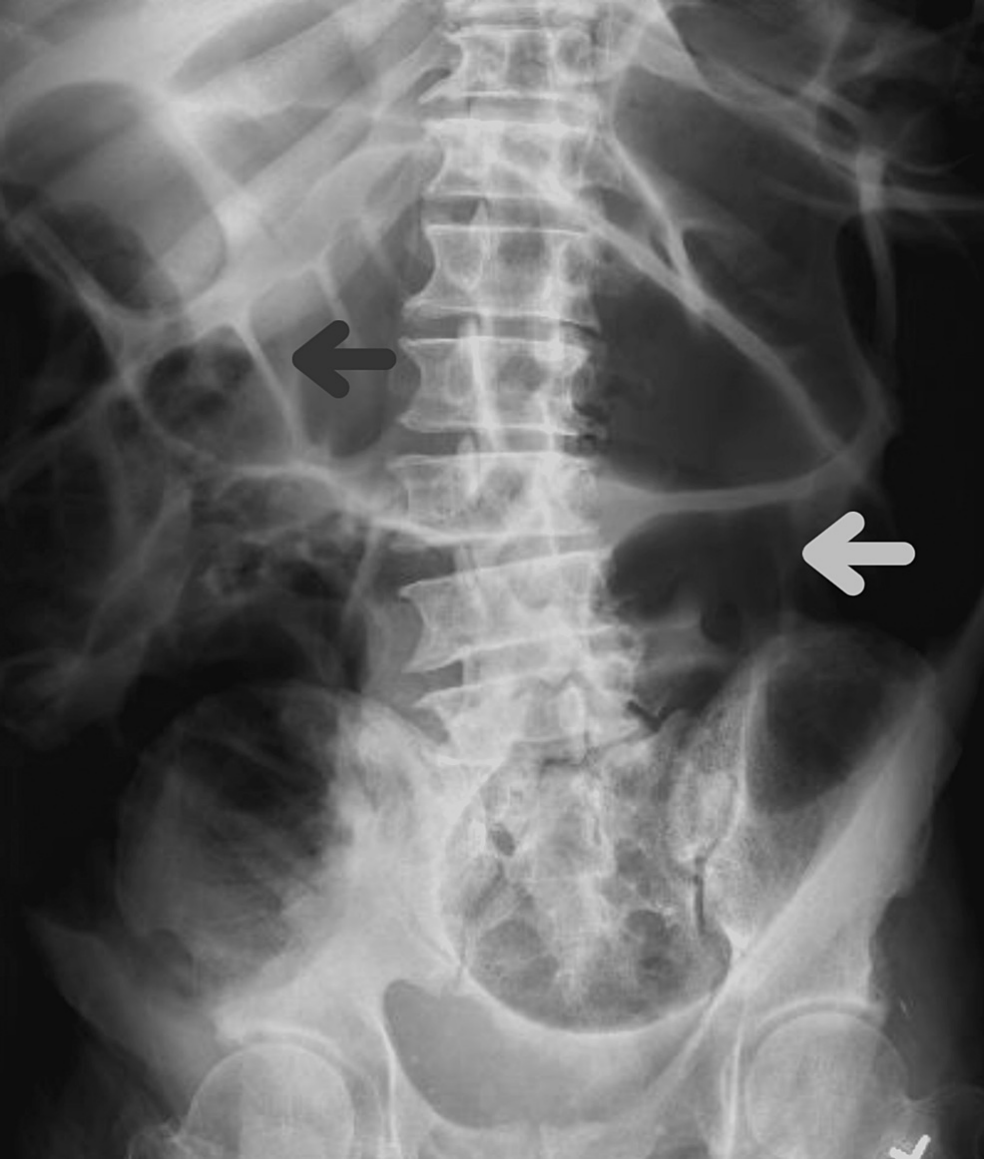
X-ray of the abdomen in flat and upright position. There are numerous distended bowel loops throughout the visualized abdomen. Limited evaluation for intraperitoneal free air on the provided view with no abnormal calcifications.
